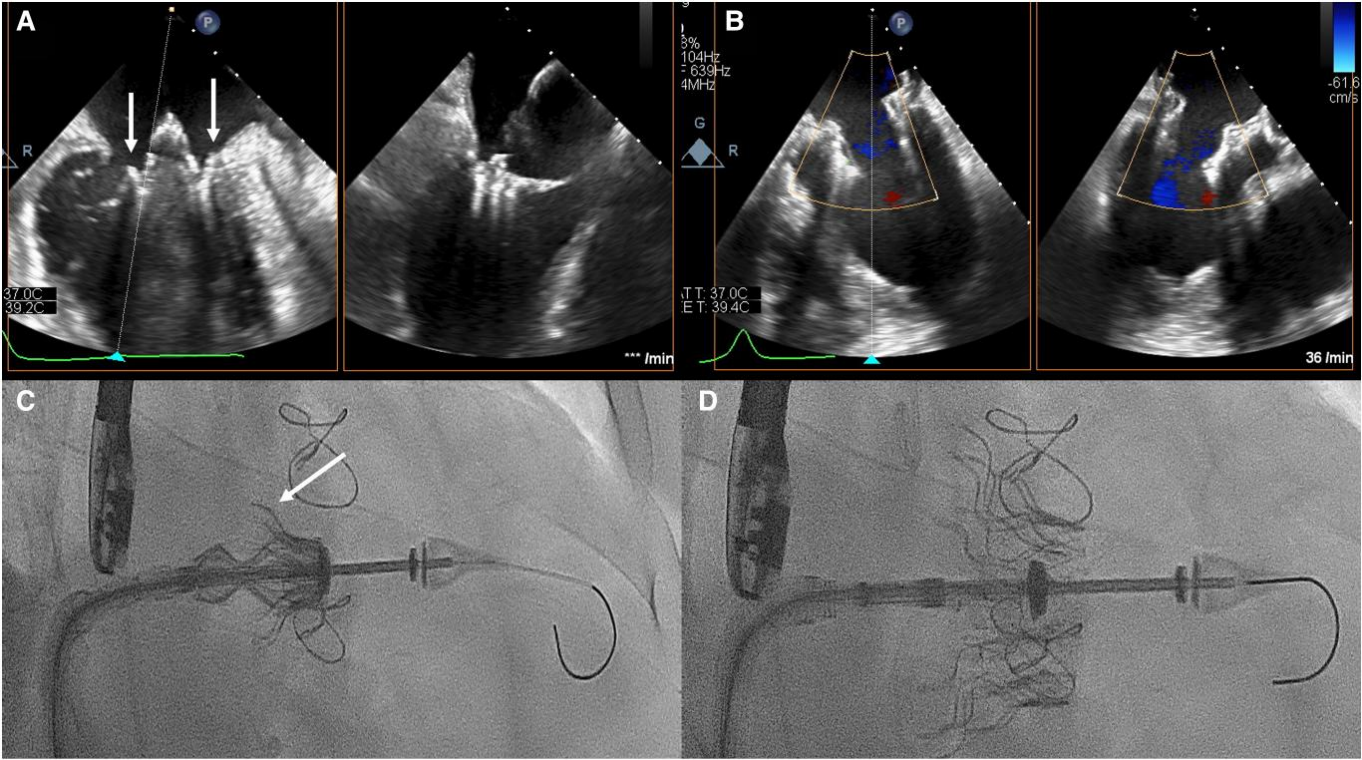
Implantation of the Cardiovalve prosthesis in mitral position. (*A*) Transoesophageal echocardiography in midesophageal commissural view of MV with opening of the ventricular legs (arrows); (*B*) transoesophageal echocardiography midesophageal commissural view of mitral valve final result without any mitral regurgitation; (*C*) fluoroscopy (projection RAO 26° and caudal 12°) showing open ventricular legs (arrow); (*D*) fluoroscopy (projection RAO 26° and caudal 12°) after final implantation.
